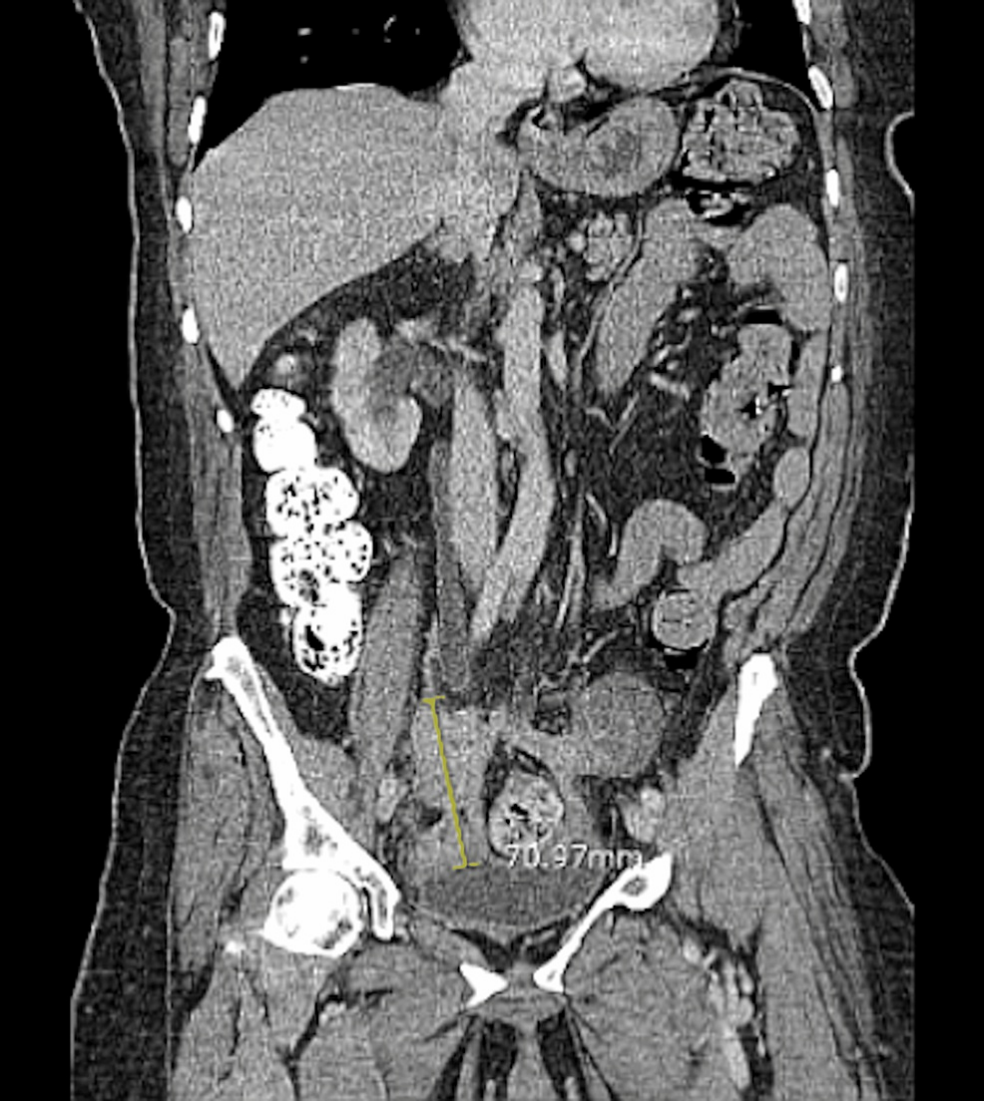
CT scan shows a cecum mass (coronal).
